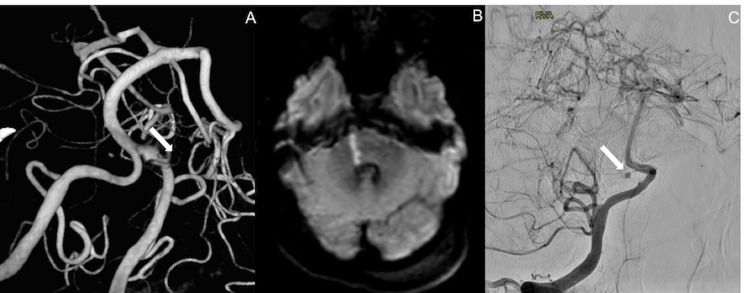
Ruptured basilar perforator aneurysm and infarct. Three-dimensional rotation angiography (A) demonstrates the focal contrast pooling, which was not seen on the initial angiogram. MRI shows a small basilar perforator stroke (B). A follow-up angiogram (C) confirms the presence of a basilar artery perforator aneurysm.
